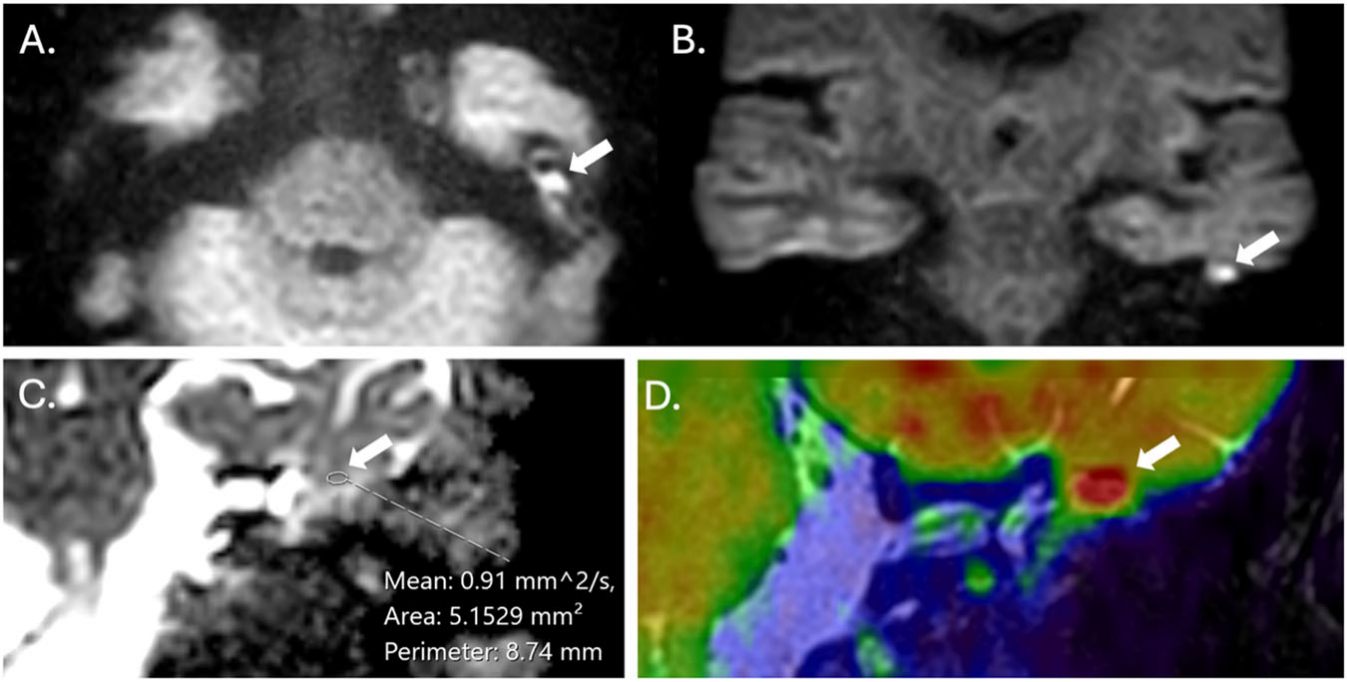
MRI of a left-sided middle ear cholesteatoma. Axial (A) and coronal (B) non-EPI DWI sequences through the temporal bones of a patient with a left posterior epitympanic cholesteatoma (arrows). The relationship with the middle cranial fossa is better depicted in the coronal sequence. Coronal ADC map (C) demonstrating restricted diffusion at the site of the cholesteatoma measured using an elliptical ROI (arrow). Coronal colourised coronal MRI fusion (between HASTE DWI MRI and T2 SPACE sequences) (D) highlighting the position of the cholesteatoma (arrow) relative to the lateral semicircular canal and tegmen tympani
